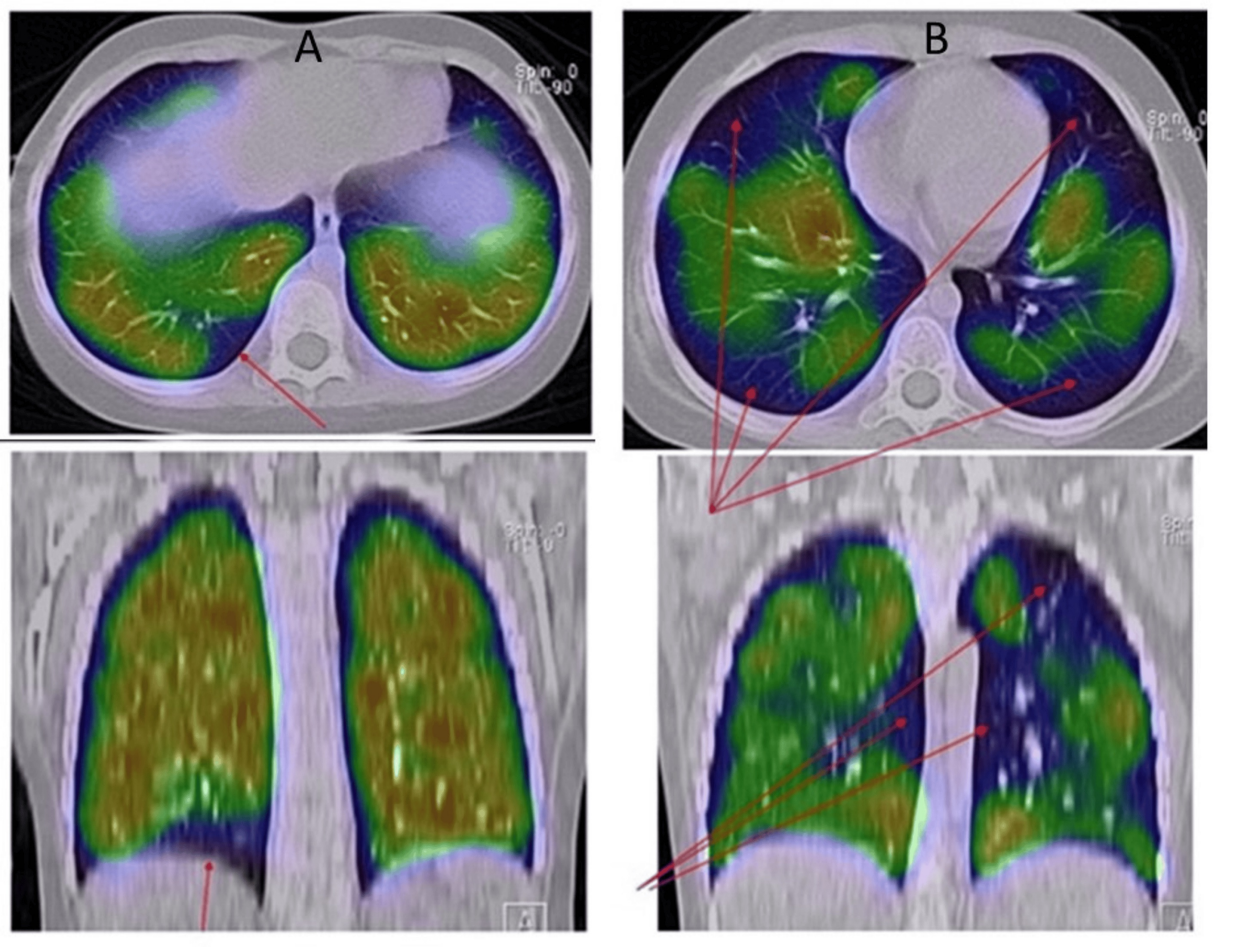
Pulmonary ventilation-perfusion scintigraphy of Case 1: (A) first episode and (B) second episode
